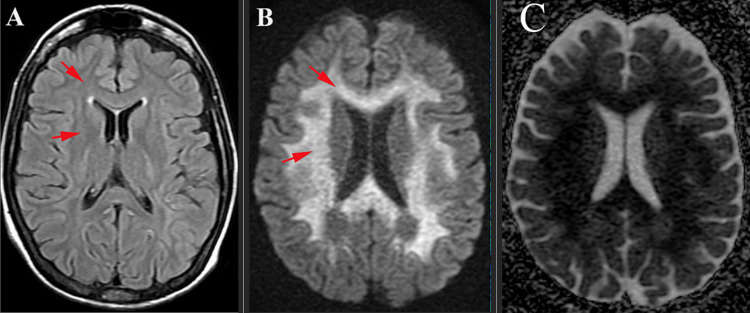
MRI of the patient performed approximately 5 hours post-seizure episodes. (A) Axial FLAIR sequence revealing hyperintensity of the white matter of the bilateral cerebral hemisphere in the frontoparietal region (red arrows), the corpus callosum, the internal capsules, and the cerebellar peduncles. (B) Diffusion-weighted magnetic resonance imaging demonstrates restricted diffusion on diffusion-weighted imaging (DWI) (red arrows). (C) Apparent diffusion coefficient (ADC) values were found to be 0.310-0.550 in affected white matter and 0.880 in unaffected white matter. FLAIR: fluid-attenuated inversion recovery
